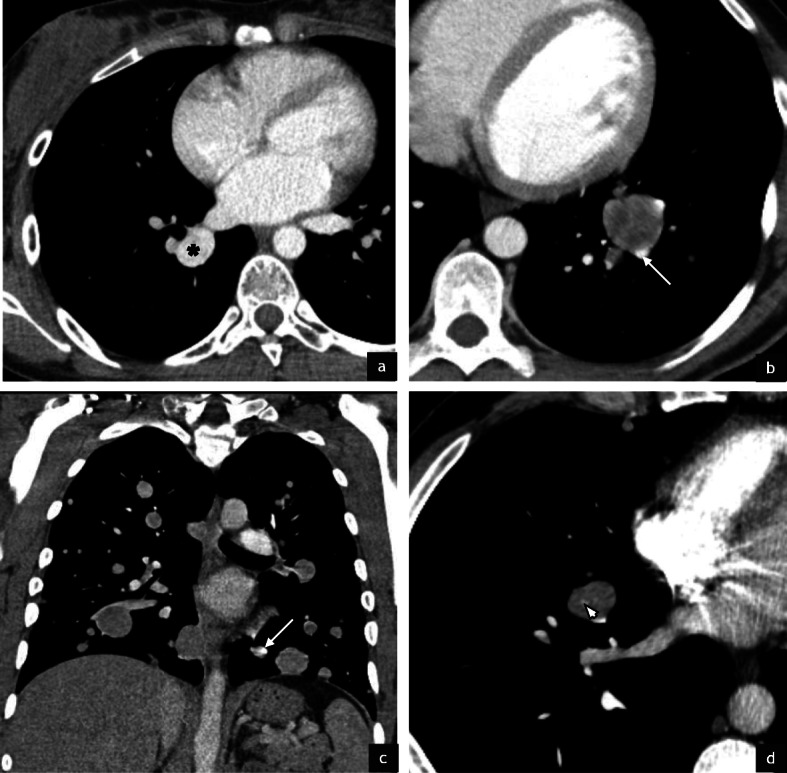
Pulmonary metastatic disease in ASPS. Post contrast axial CT in a 22 year old female (a) demonstrates a hyperdense right lower lobe pulmonary metastasis (a, black asterisk) which is almost isodense to the pulmonary vasculature. Post contrast CT in a 51 year old male (b) and a 29 year old male (c) shows prominent feeding vessels to multiple pulmonary metastases (b,c white arrows). Despite the small size of the metastases, intratumoural vessels can also be observed as in this post contrast CT of a 24 year old male patient (d, white arrowhead)
